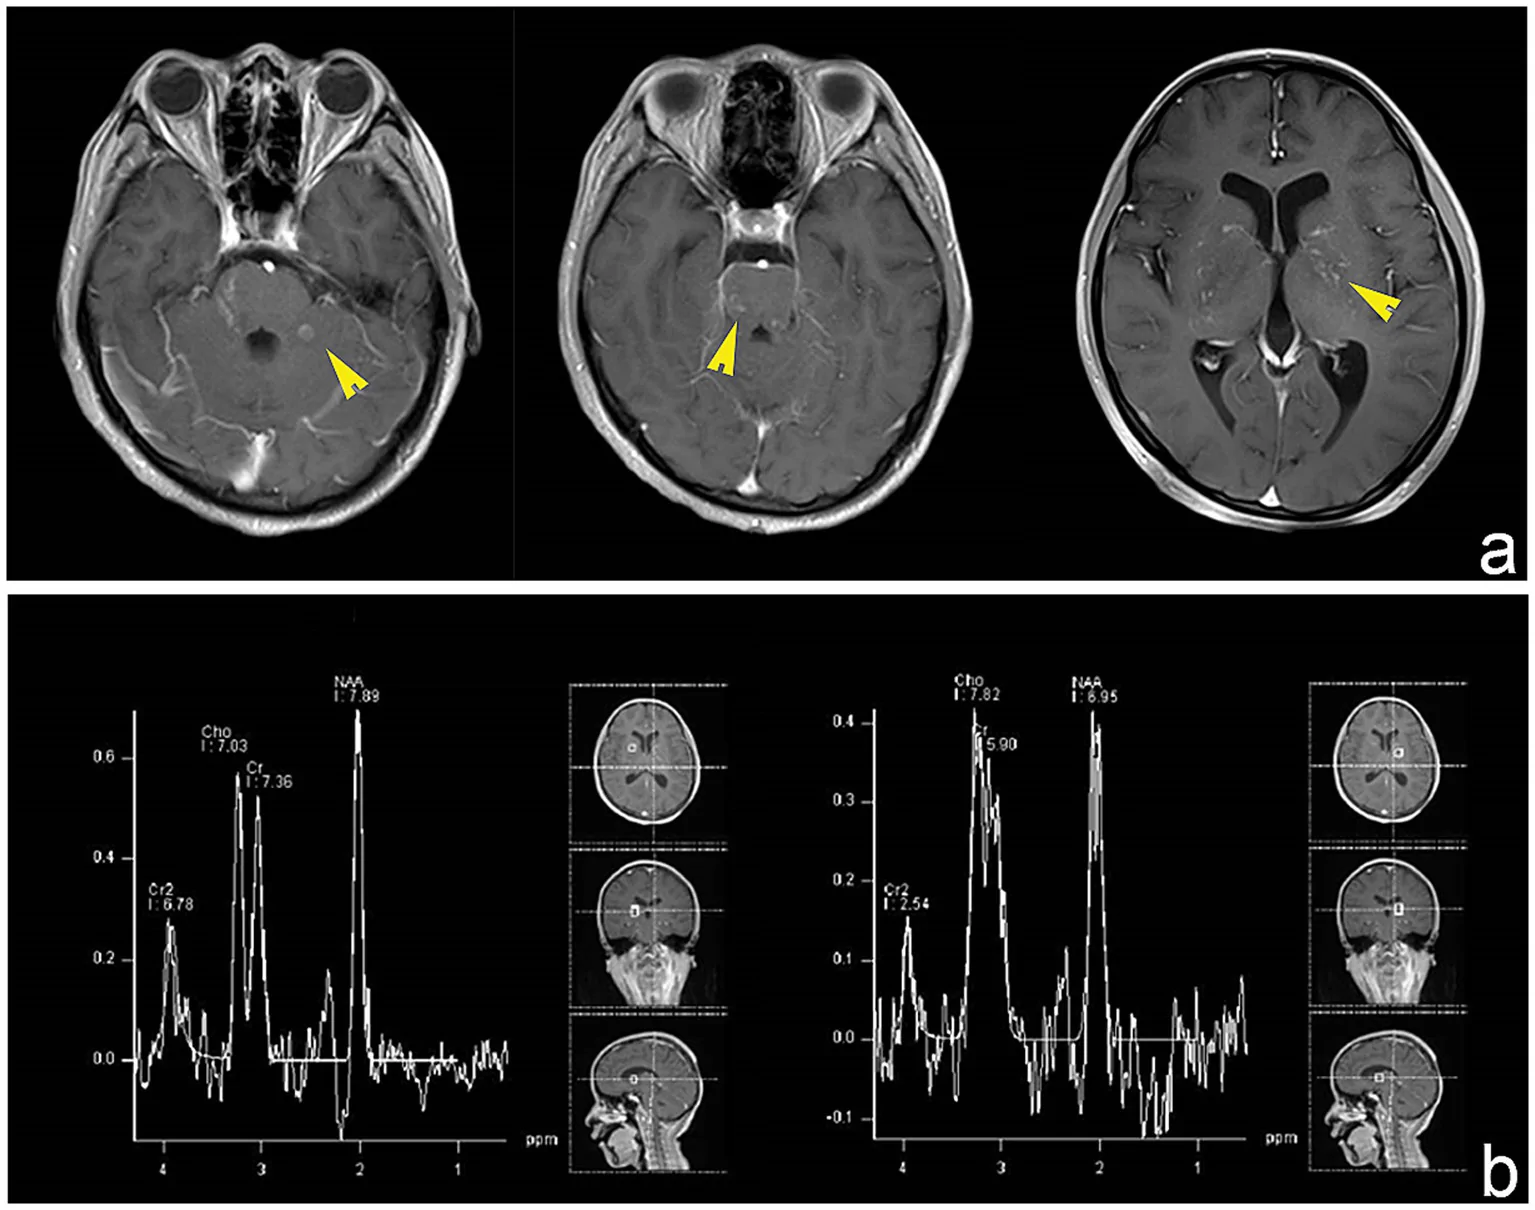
Contrast-enhanced brain MRI and MRS of the patient (a) Contrast-enhanced brain MRI showed multiple punctate and irregular abnormal enhancing lesions (yellow arrows). (b) MRS indicated a significant decrease in N-acetylaspartate (NAA)/creatine (Cr) ratio and an increased choline (Cho)/Cr ratio in the lesion area.
